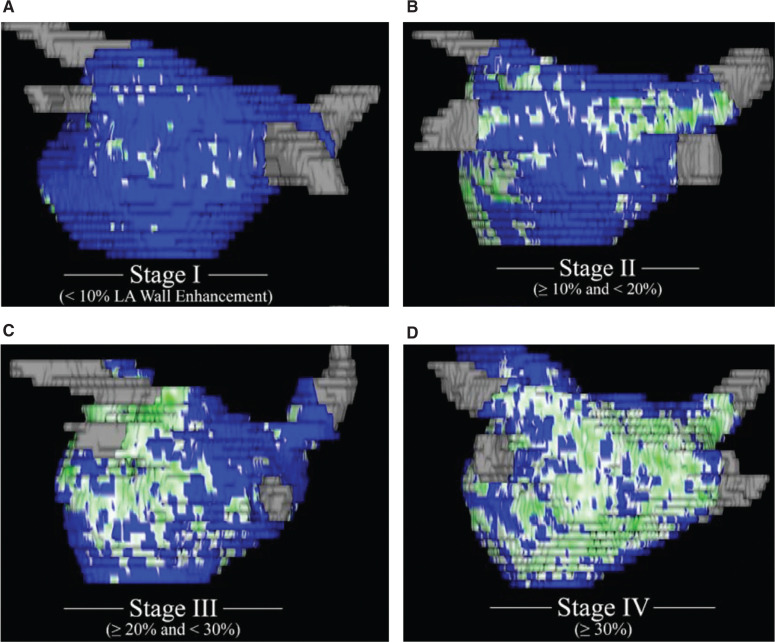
Progression of fibrosis in the posterior LA in patients with PRAF as confirmed by 3D delayed-enhancement magnetic resonance imaging scan. A: Stage 1 (< 10% of the atrial wall). B: Stage 2 (≥ 10% to < 20% of the atrial wall). C: Stage 3 (≥ 20% to < 30% of the atrial wall). D: Stage 4 (≥ 30% of the atrial wall).
